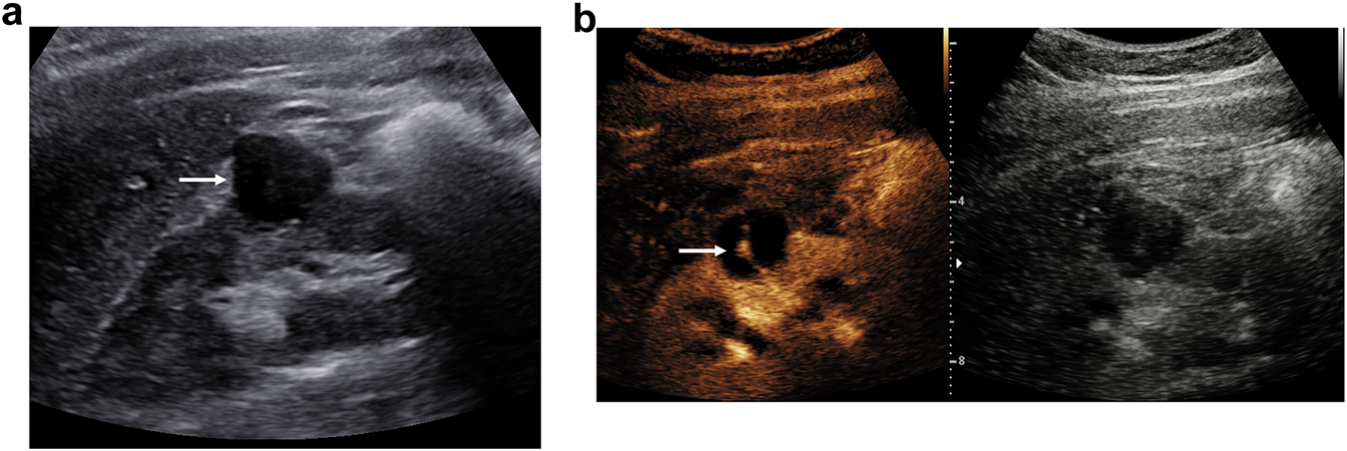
A 78-year-old male patient with ‘acute kidney injury’, undergoing a routine examination of the kidneys. a On the B-mode ultrasound examination, there is an 18-mm cystic structure at the mid aspect of the right kidney, with some debris within. b An immediate CEUS examination, which is safe in the presence of renal impairment, using 1.2 mL of SonoVue™ (Bracco SpA, Milan), there is enhancement of a > 2-mm septation (arrow) within the cyst, classifying this a Bosniak type IIF
